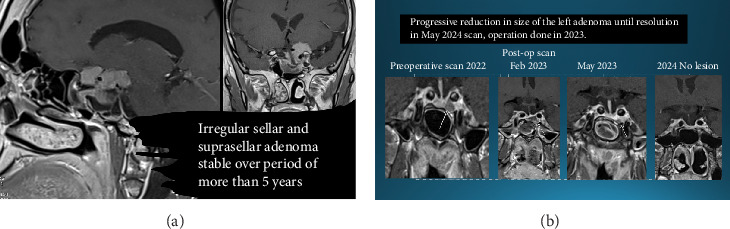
(A) MRI May 2023. (B) MRI May 2024.
